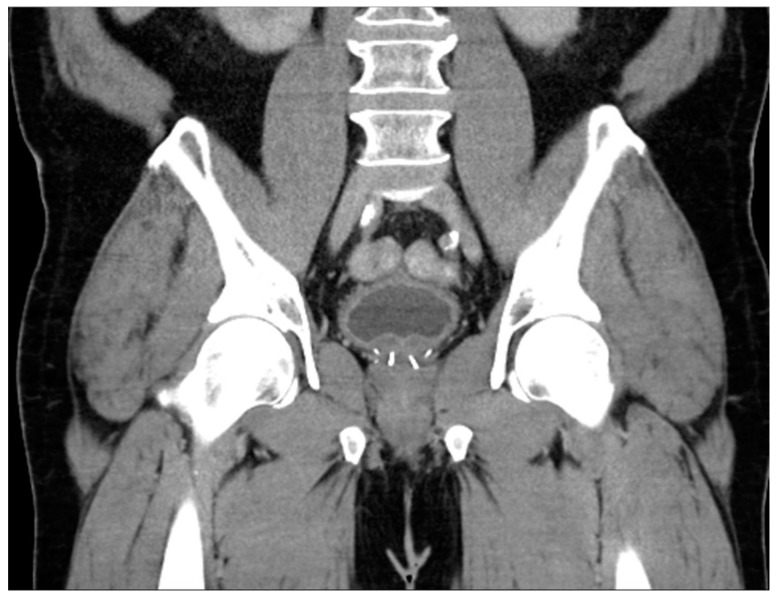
Coronal CT appearance of UroLift device indicated by linear densities within the bilateral prostate gland.
